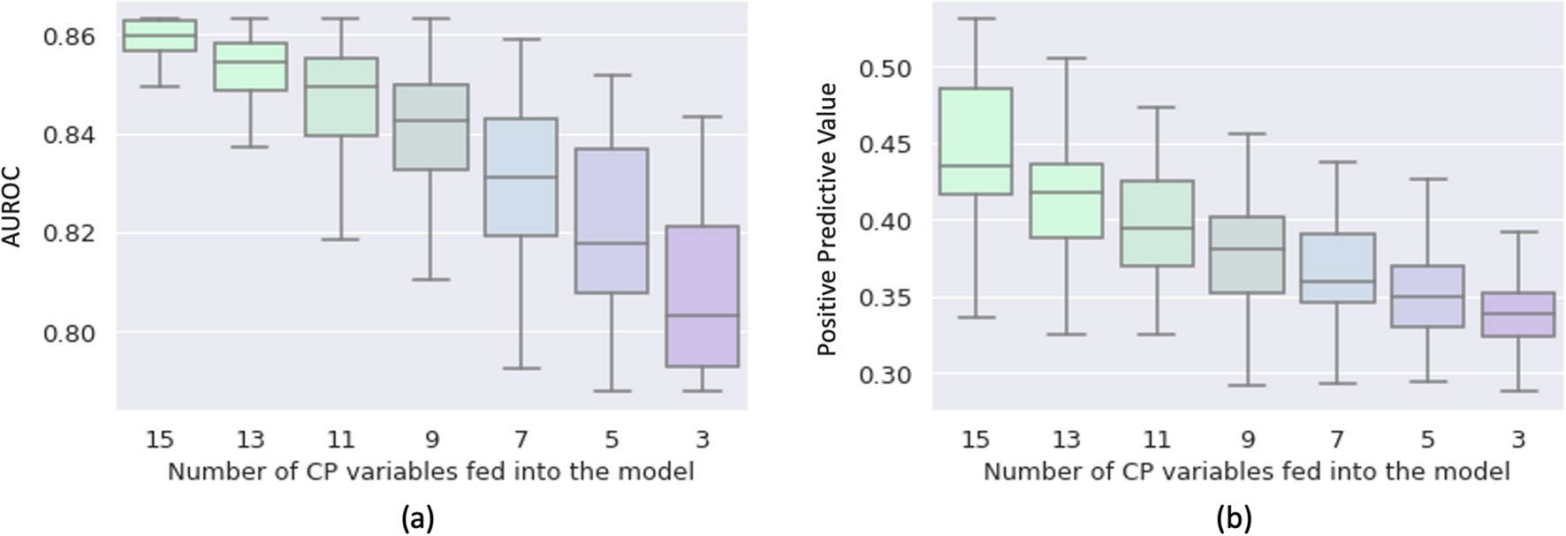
Performance of MeTra in terms of the AUROC values (a) and the positive predictive values (b) as a function of the number of clinical parameters available to the model. The x-axis denotes the number of clinical parameters fed into the model alongside the chest radiograph. For each number of clinical parameters, the experiment was repeated 100 times with randomly chosen subsets of variables to prevent bias due to the choice of variables.
